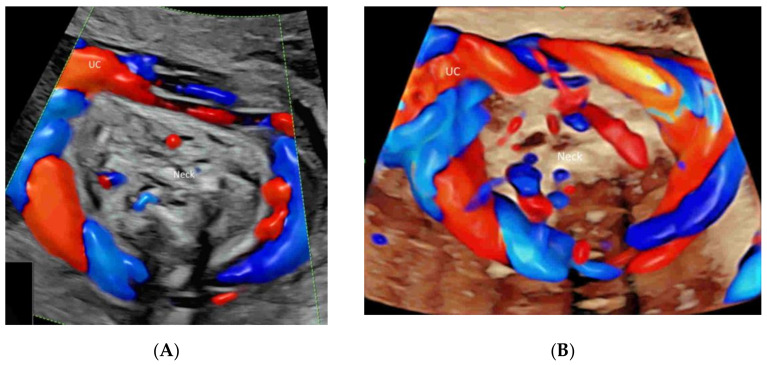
Color Doppler in a transverse plane of the neck of a fetus at 24 weeks’ gestation showing nuchal cord (A) with the umbilical cord (UC) wrapping around the neck. A spatiotemporal image correlation volume acquisition is displayed in glass-body mode (B).
